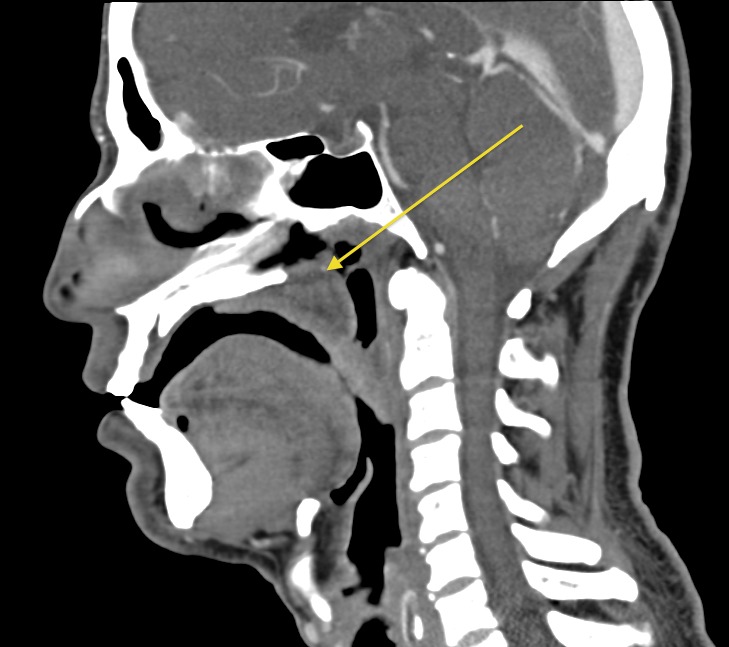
CT neck with contrast in sagittal plane. Heterogeneous soft tissue is present in the nasopharynx.
